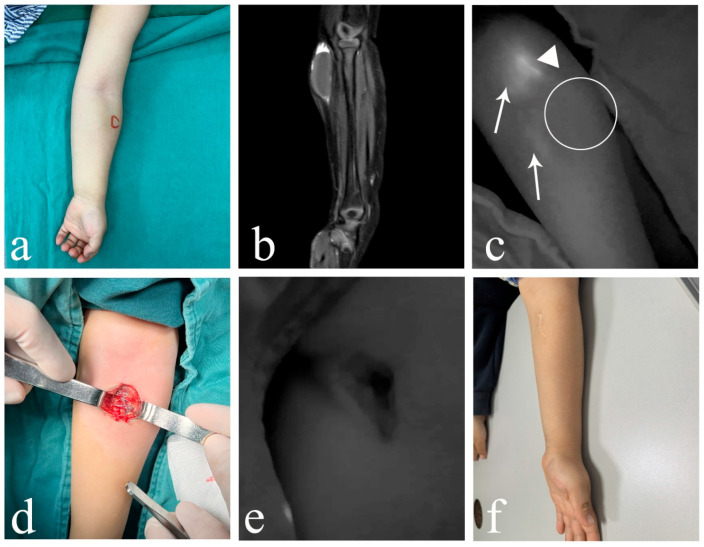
A 2-year-old male patient suffered from macro cLM with ICH in left forearm. (a) Clinical appearance preoperatively. (b) Preoperative MRI indicated macro cysts with ICH. (c) ICG lymphography showing that one lymph vessel on the ulnar side of the left forearm bypassed the lesion and flowed into the cubital fossa lymph node. No communication between lymph vessel and the lesion cite was observed. (d) Gross observation of ICH intraoperatively. (e) No ICG was found inside the lesion. (f) Twelve months postoperatively, complete regression of cLM met the criterion of excellent curative effect. *Arrow head*, lymph vessel; *circle*, region of the lesion; *triangle*, lymph node. cLM, cystic lymphatic malformation; ICH, intracystic hemorrhage; MRI, magnetic resonance imaging; ICG, indocyanine green.
